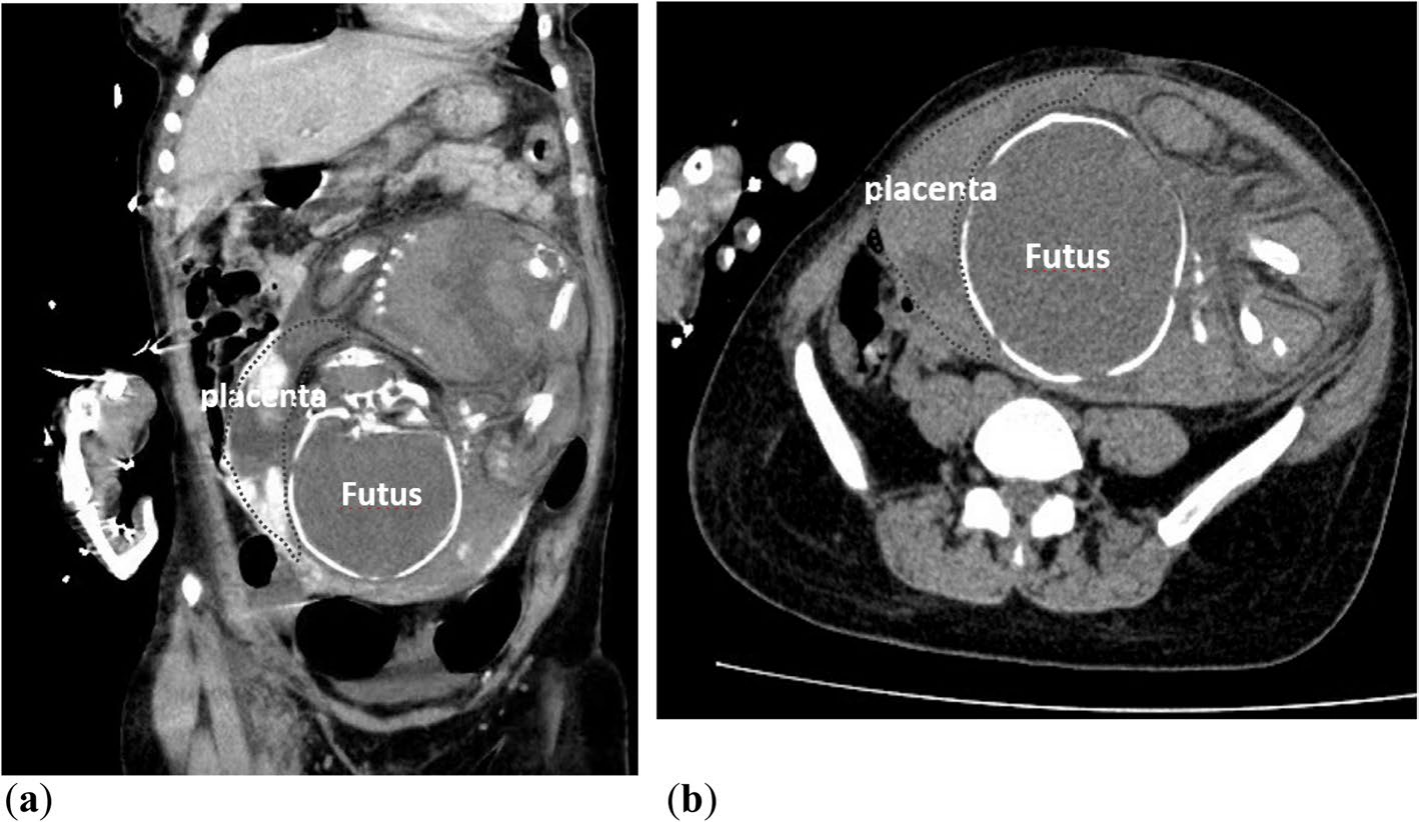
Sagittal- or coronal-view CT revealing crescent placenta
